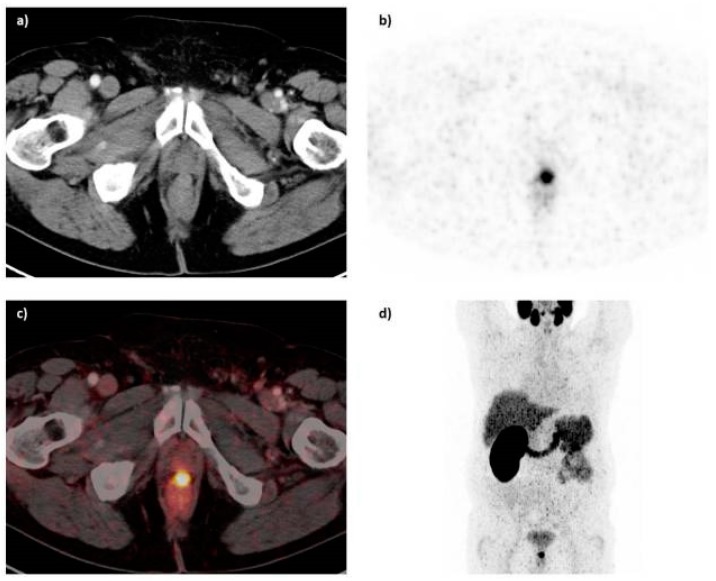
^68^Ga-PSMA-11 PET/CT image of a patient with locally recurrent prostate cancer (PSA 3.7 ng/mL) after radical prostatectomy (SUVmax 12.4) who received 140 MBq of the ^68^Ga-labeled tracer molecule and was scanned at 1 h p.i.; (a) CT image; (b) PET image; (c) PET/CT fusion image; (d) MIP (Reprinted with permission of [145]).
